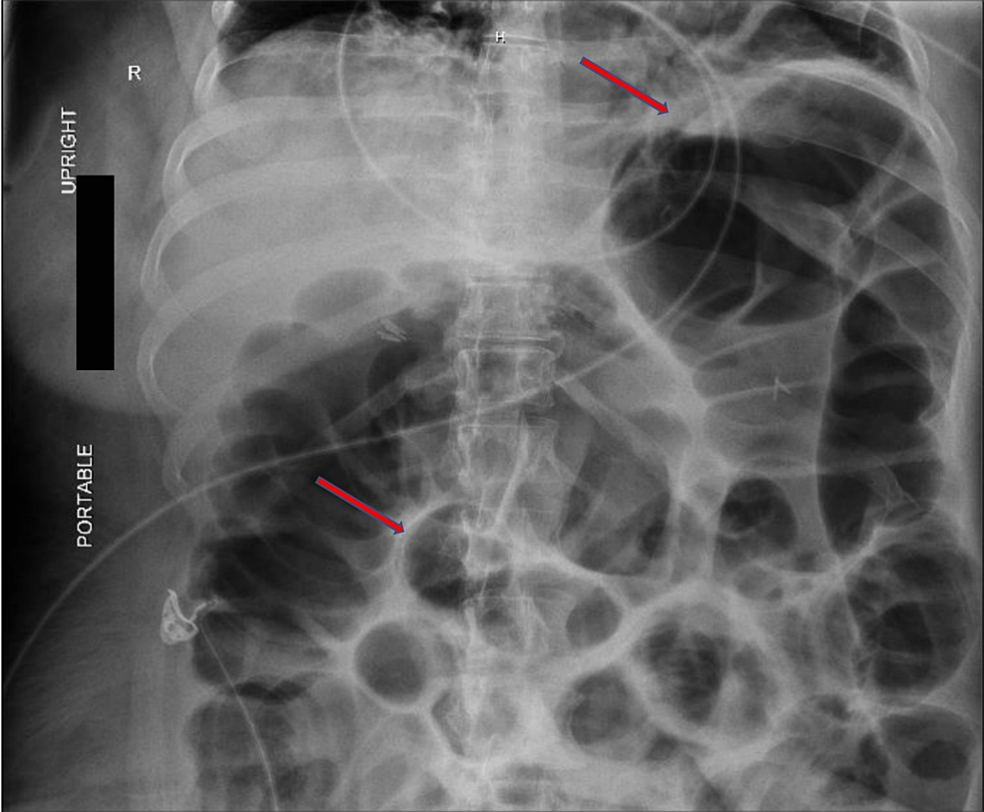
Abdominal X-ray demonstrating small and large bowel distention (red arrows).
